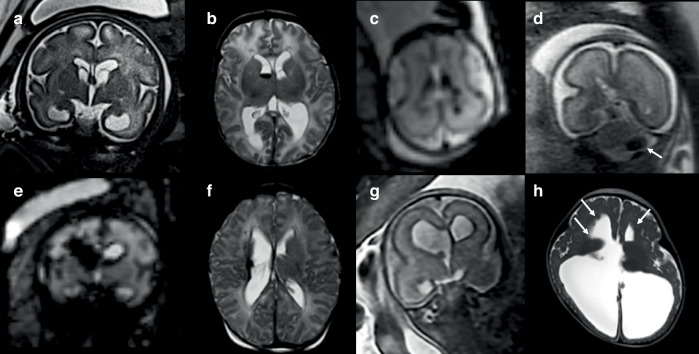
Intracerebral hemorrhage on fetal MRI. Coronal T2-weighted MRI (a) at 33 gestational weeks showing mild ventriculomegaly with cysts at the bilateral caudothalamic grooves, and postnatal axial T2-weighted MRI in this case (b) showing small germinal matrix hemorrhage, septation in the right lateral ventricle and white matter hyperintensities due to congenital CMV. Axial echoplanar imaging (EPI) at 22 gestational weeks (c) in monochorionic twin showing bilateral germinal matrix-intraventricular hemorrhage (GMH-IVH), with cerebellar hemorrhage (d) on coronal T2-weighted MRI. GMH-IVH with right periventricular venous hemorrhagic infarction (e) at 31 gestational weeks due to *COL4A2* variant, and postnatal axial T2-weighted MRI showing chronic changes (f). Twin with ventriculomegaly on coronal T2-weighted MRI (g) at 22 weeks and bilateral GMH confirmed on EPI (not shown), with postnatal follow-up MRI at 8 months (h) showing persistent severe ventriculomegaly despite ventriculoperitoneal shunting for post-hemorrhagic hydrocephalus with parenchymal volume loss and periventricular nodular heterotopia.
